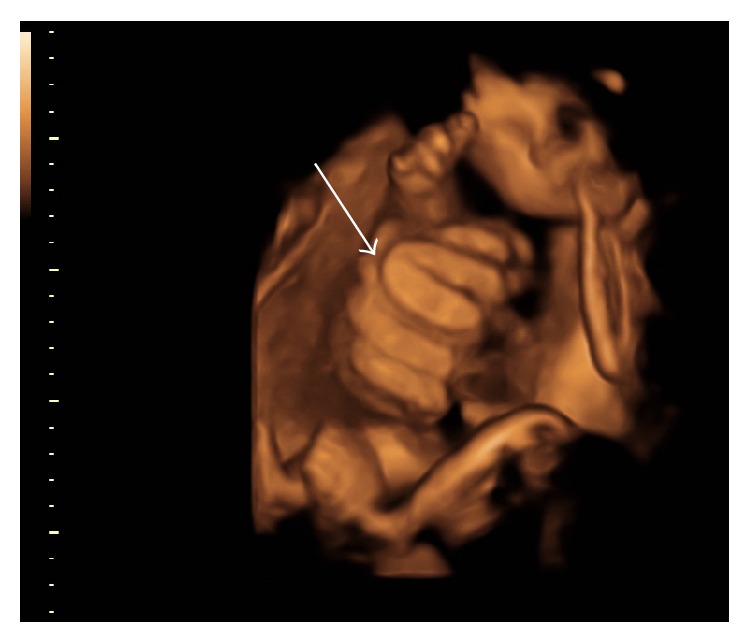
3D ultrasound image demonstrating free loops of intestine in amniotic cavity at 34 weeks.
